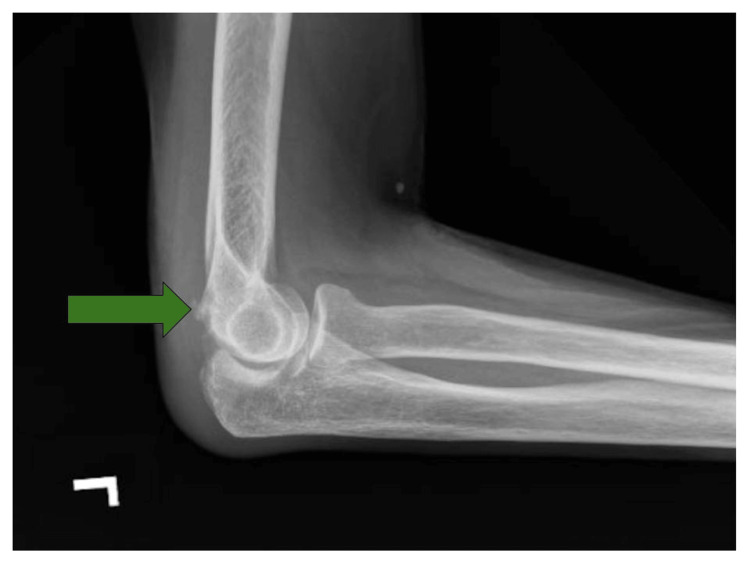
X-ray of the left elbow Slight spurring is noted along the dorsal aspect of the distal humerus (green arrow) which could be a sequela of prior injury or related to heterotopic ossification given history.
